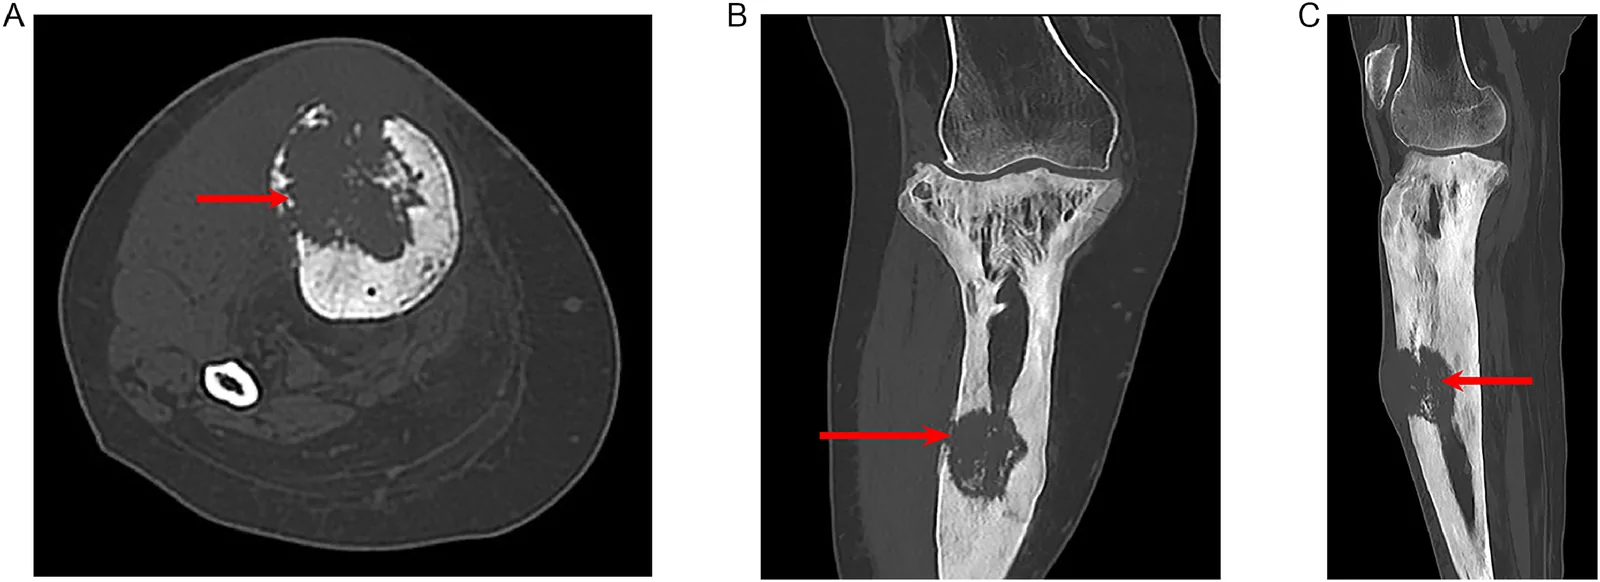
Axial (A), coronal (B), and sagittal (C) CT images demonstrate a lytic lesion in the proximal to mid tibial diaphysis with cortical destruction and soft tissue component anteriorly (arrow). There is diffuse cortical thickening of the tibia as well as trabecular thickening of the proximal tibia consistent with underlying Paget’s disease.
